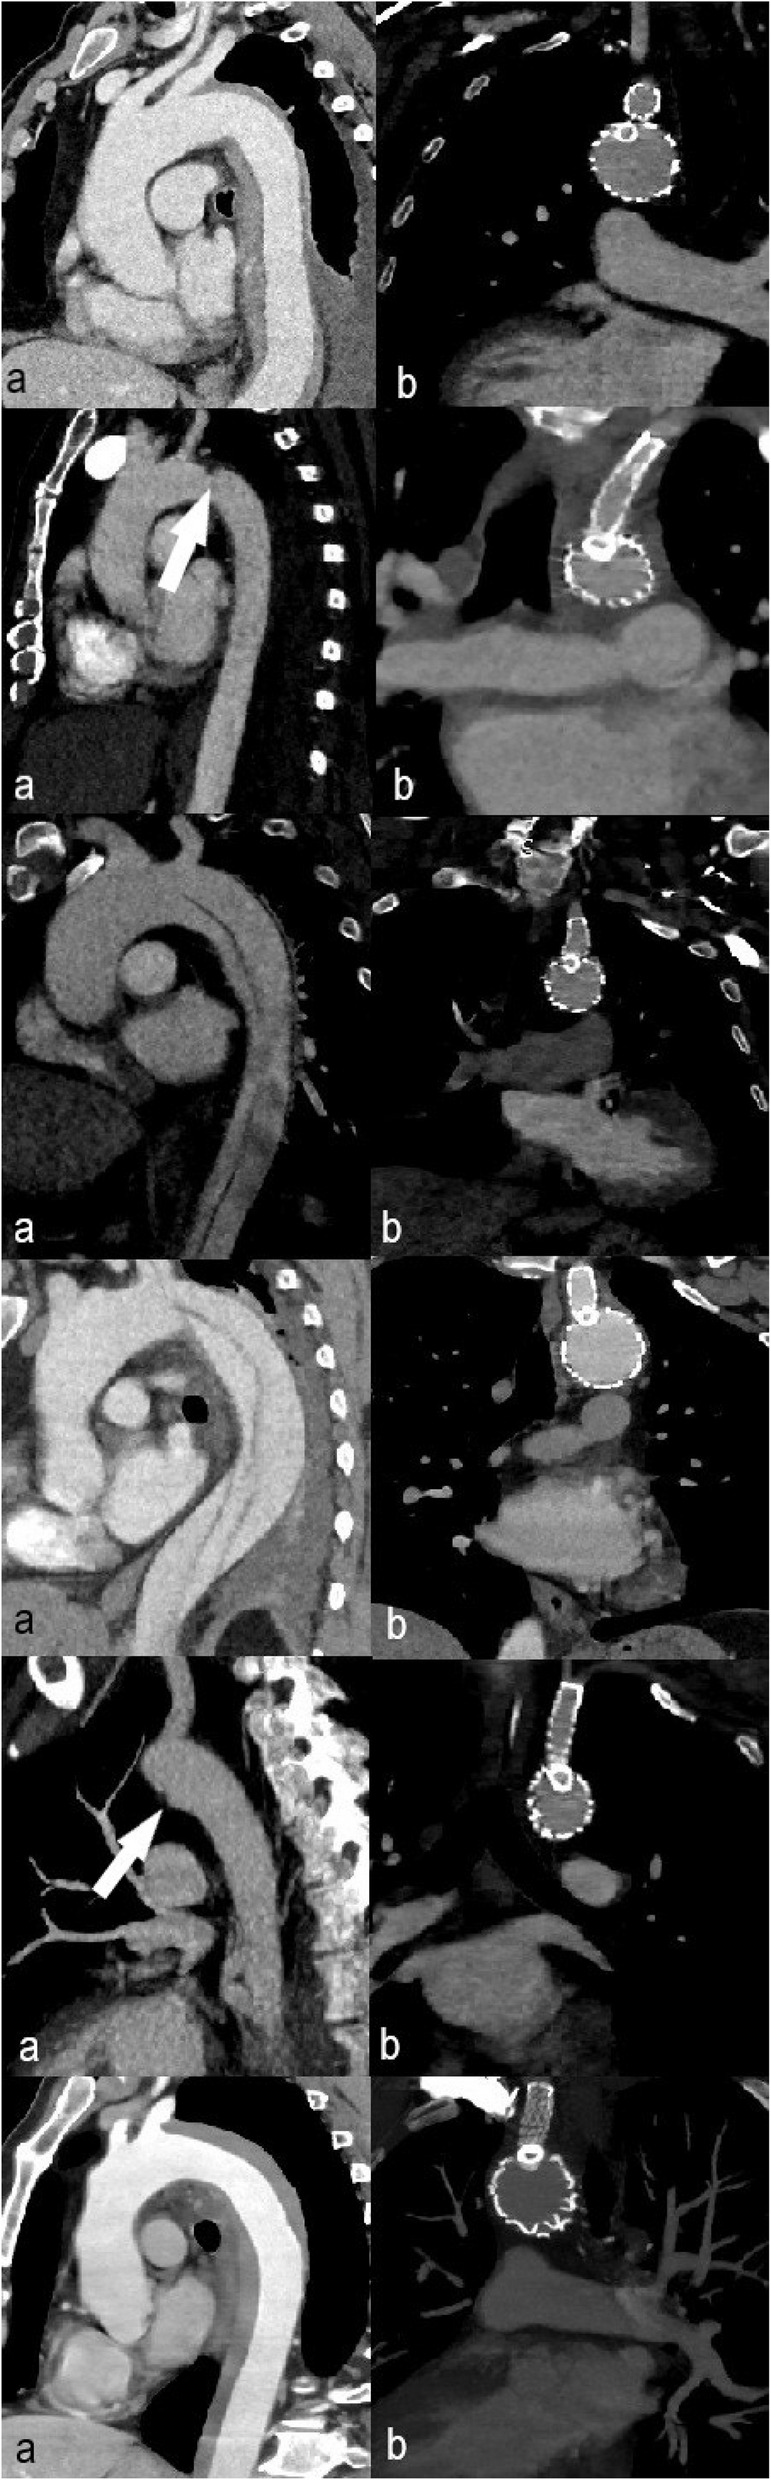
a Pre-procedural reconstructed CTA images and (b) 6-months post-procedural reconstructed CTA images. Each row represents an individual patient treated in our center. 1st row: IMH, 2nd row: PAU (white arrow, a), 3rd row: TBAD, 4th row: TBAD, 5th row: TAI (white arrow shows traumatic pseudoaneurysm, a), 6th row: IMH
